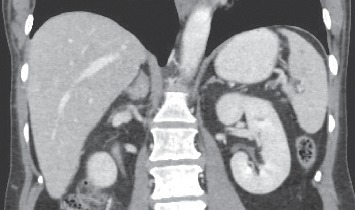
CT showed decreased multiple hypoechoic nodules in the liver and spleen.
